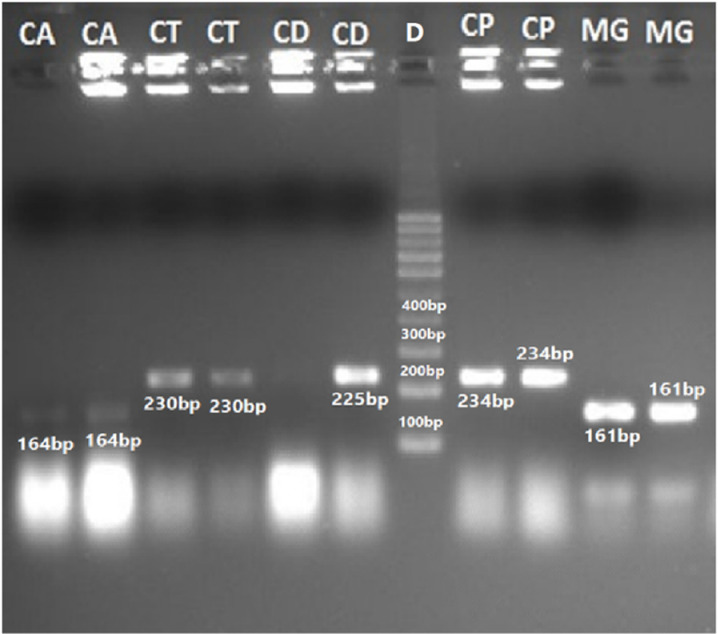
Using PLB gene species-specific primers PCR amplified product of CA=C. albicans (164 bp), CT=C. tropicalis (230 bp), C. dubliniensis (225 bp), C. parapsilosis (234 bp), Meyerozyma guilliermondii (161 bp) and 100 bp DNA ladder (D).
